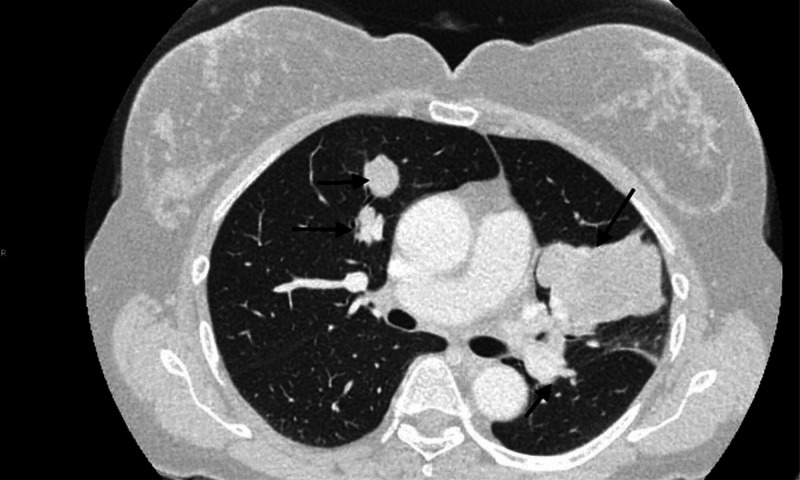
Computed tomography of the chest showing a significant progression of disease in lungs (black arrow) two months after starting everolimus and prior to starting nab-sirolimus.
